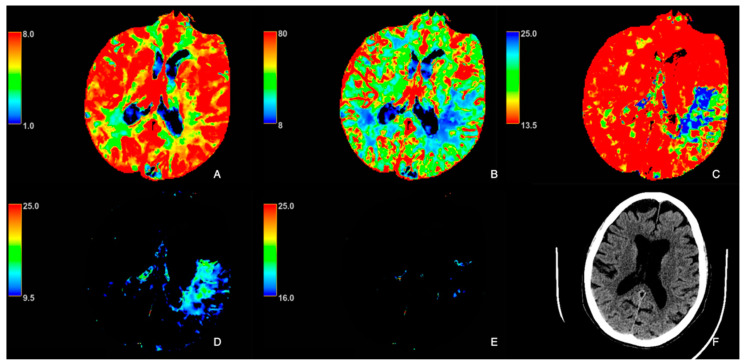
A 74-year-old female patient with acute ischemic stroke due to left middle cerebral artery occlusion. (A): Cerebral blood volume (CBV); (B): cerebral blood flow (CBF); (C): mean transit time (MTT); (D): $T_{max}^{9.5-25s}$; (E): $T_{max}^{16-25s}$; (F): axial CT brain. NIHSS onset: 17.
